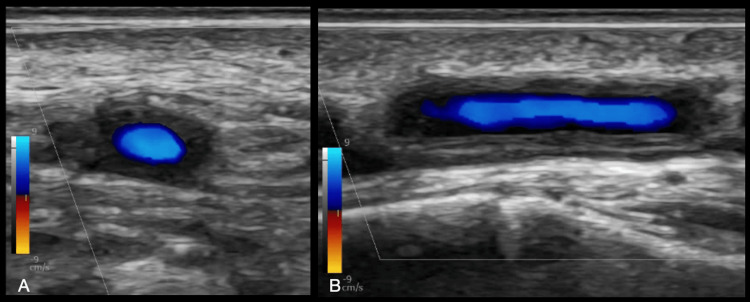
Halo sign in TAUS; hypoechoic, homogenous thickening of the superficial temporal artery. (A) transverse view, (B) Longitudinal view Image credit: Authors; consent taken from the patient with confirmed giant cell arteritis. TAUS: temporal artery ultrasound
